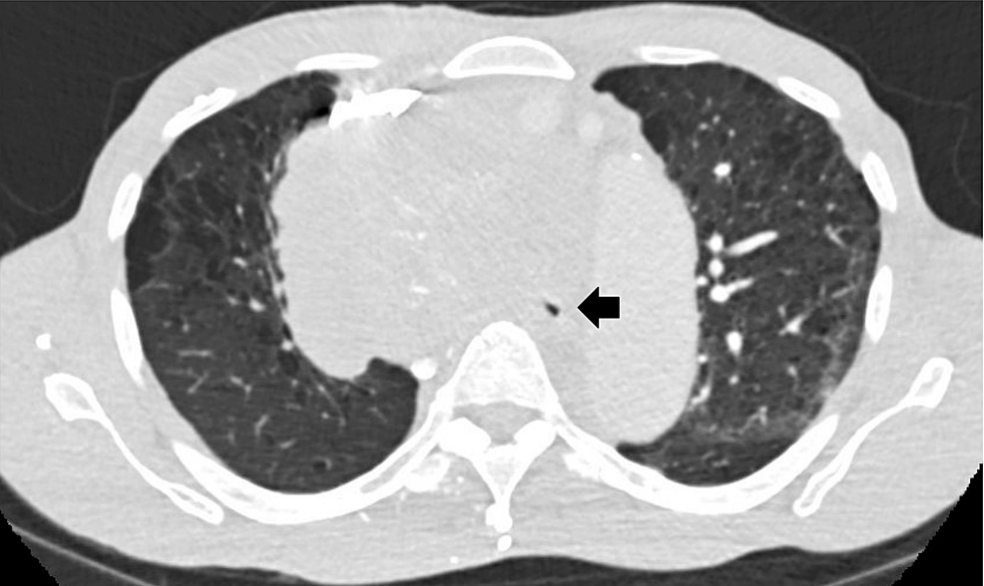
CT scan with large mediastinal mass causing critical compression of the trachea down to 4 mm diameter. The black arrow indicates a critically narrowed tracheal lumen.
